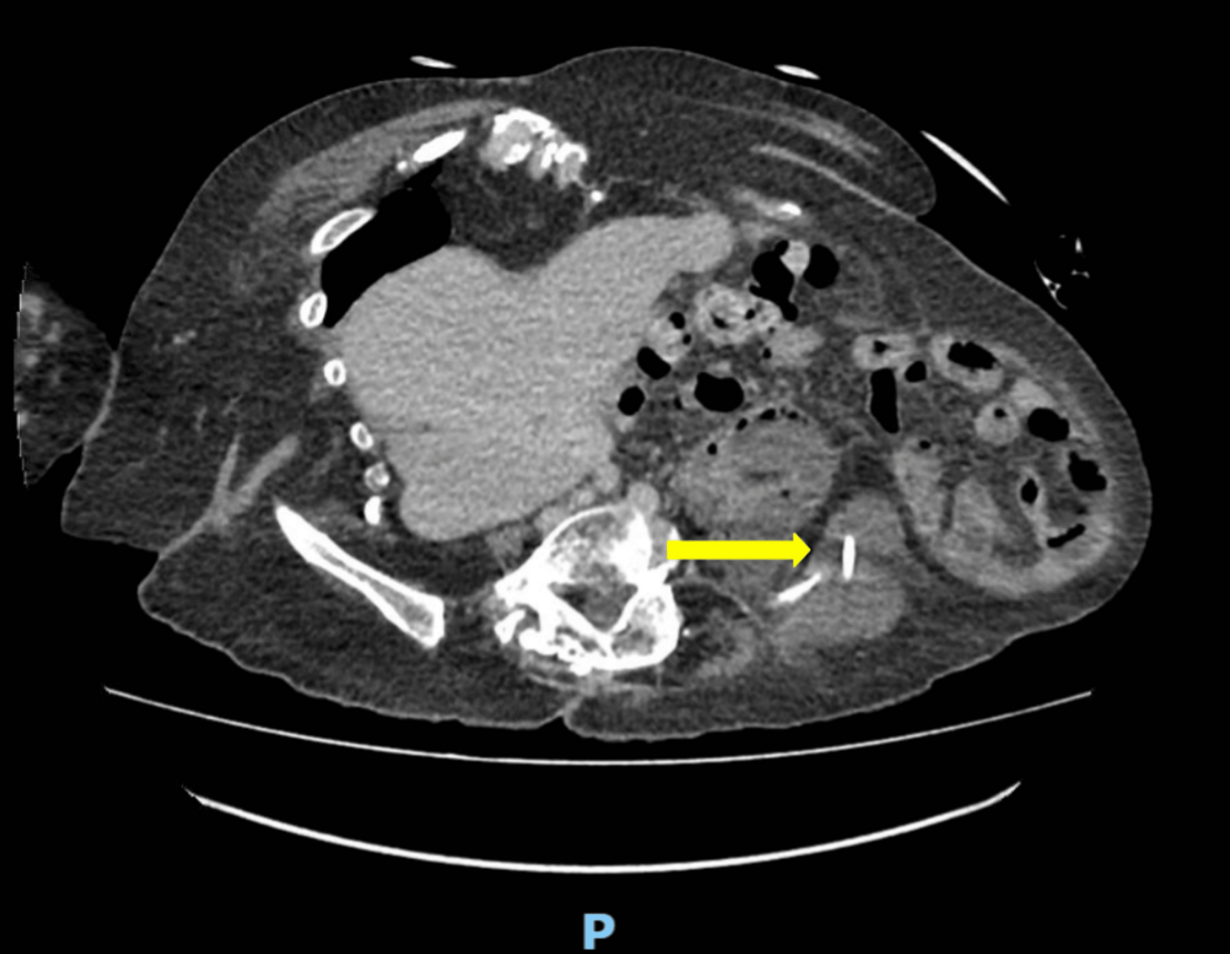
Contrast CT showing the second stent traversing the duodenal wall and extending inferolaterally (yellow arrow)
